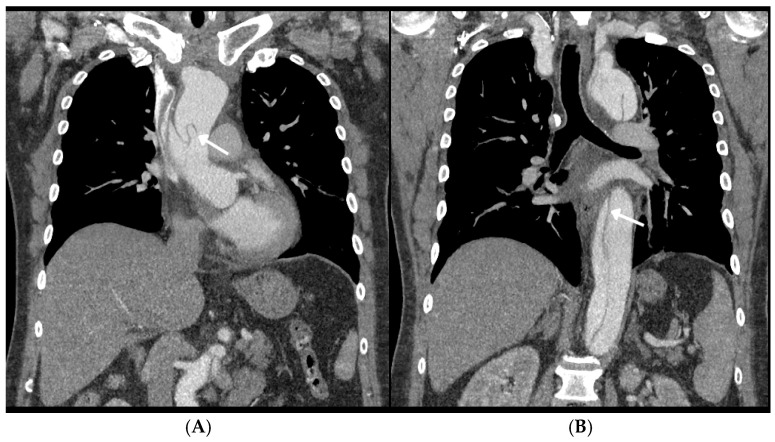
Computed tomography angiography (CTA) with the dissection of the proximal ascending (A) and descending (B) parts of the aorta (marked by arrow).
